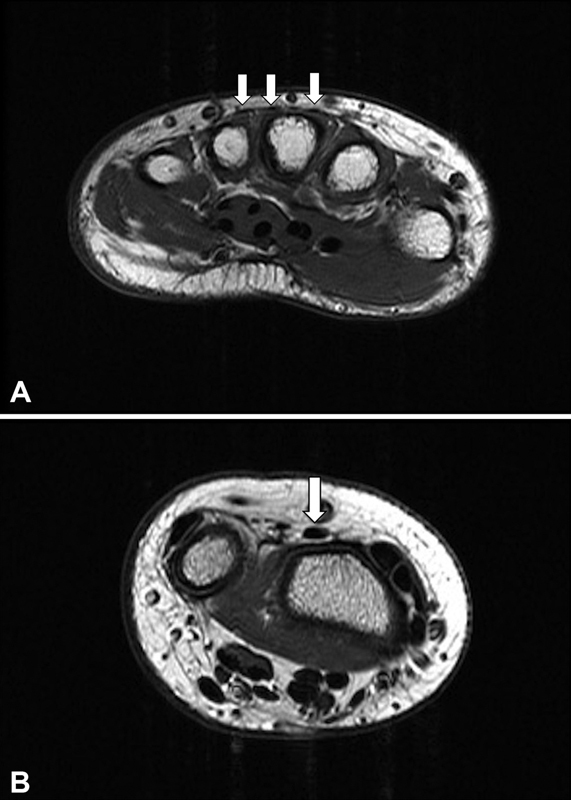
Left axial T1 magnetic resonance image. (
